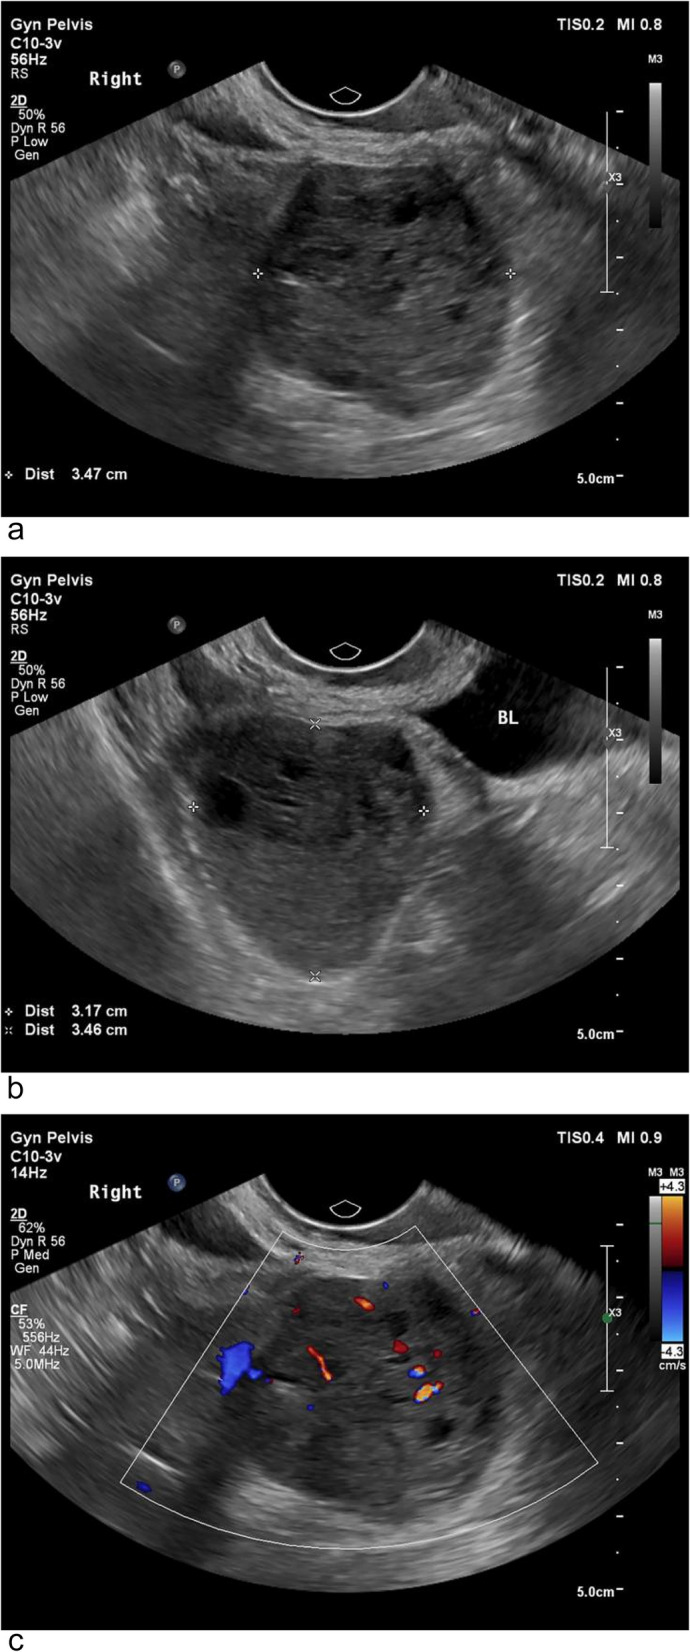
The transvaginal ultrasound at diagnosis. a and b A well-defined, hypoechoic mass is in the right adnexa, adjacent to the bladder. c Color Doppler image shows the mass contains internal flow. *BL *bladder
